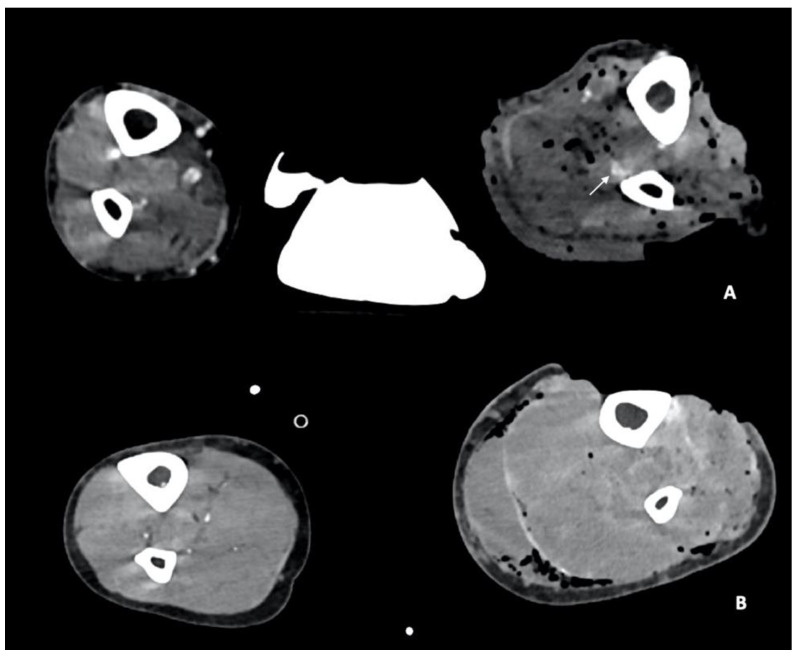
CTA, axial planes (A,B). According to Gustilo–Anderson classification, these two different patients were classified as grade IIIB (A) and IIIC (B), respectively, involving at least three of the four major systems: integument, soft tissue, bone, and nerves and vessels. In both these patients, the left lower-limb fractures are characterized by extensive bone loss, periosteal stripping with devitalized fragments, massive contamination, and poor soft-tissue coverage. In (A), the left peroneal artery contusion can be noted (arrow), while in (B), the left arteries cannot be recognized, indicating arterial injuries that require reperfusion.
